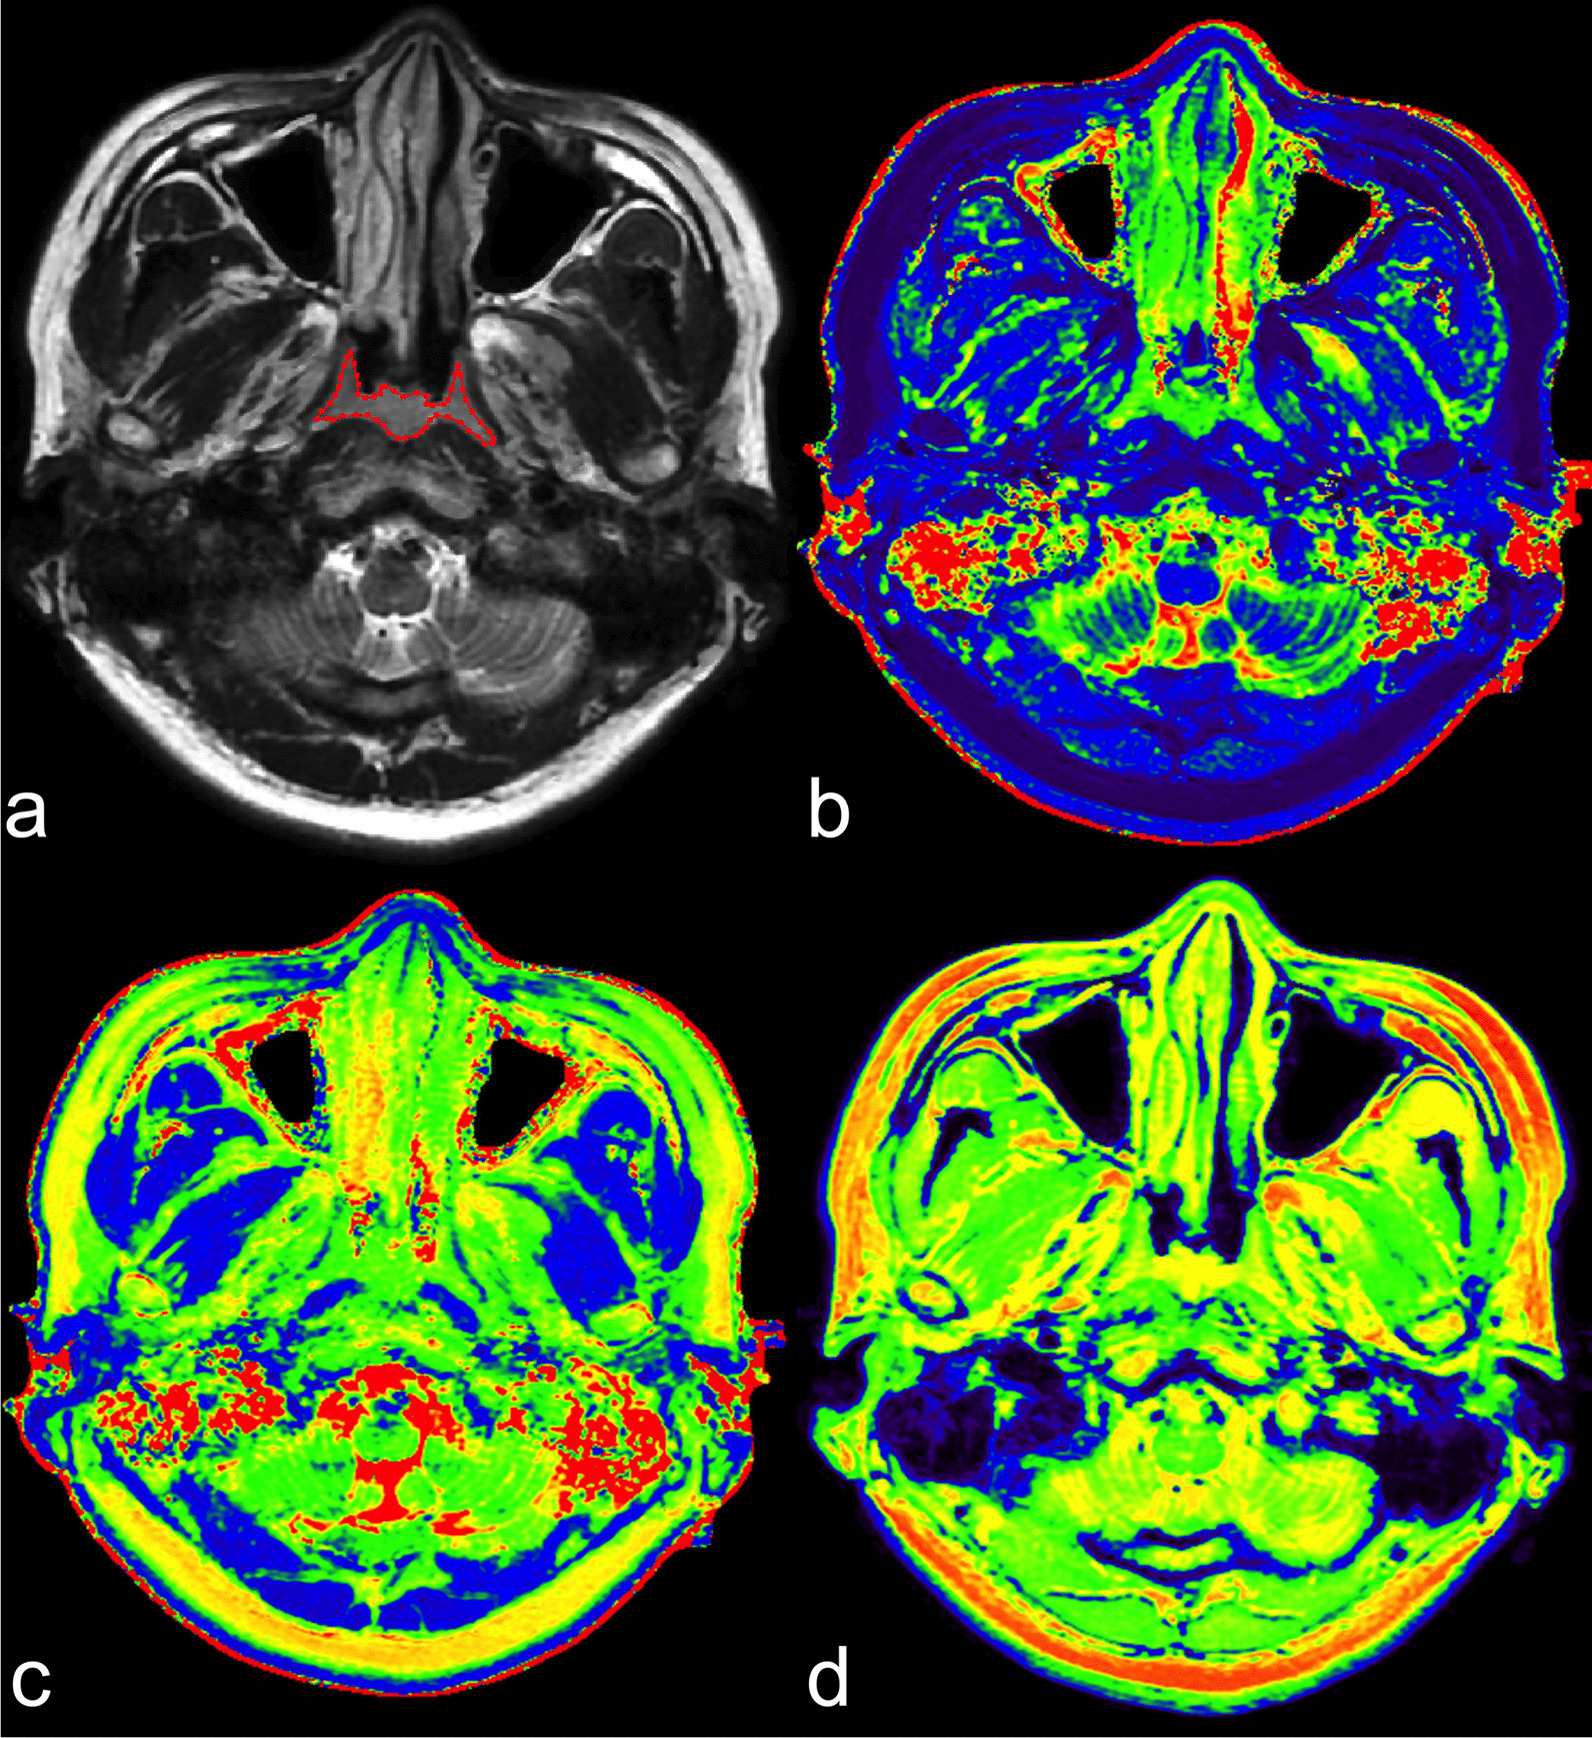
SyMRI of a 38-year-old female volunteer. a Axial T2WI shows hyperenhancing tissue along the posterior nasopharyngeal wall and bilateral lateral nasopharyngeal wall. Bilateral pharyngeal recess are collapsed and show symmetric wall thickness. VOI was manually delineated along the border of the benign mucosa slice by slice. Derived T1 map (b), T2 map (c) and PD map (d). SyMRI, synthetic magnetic resonance imaging; T2WI, T2-weighted image; VOI, volume of interest; PD, proton density
